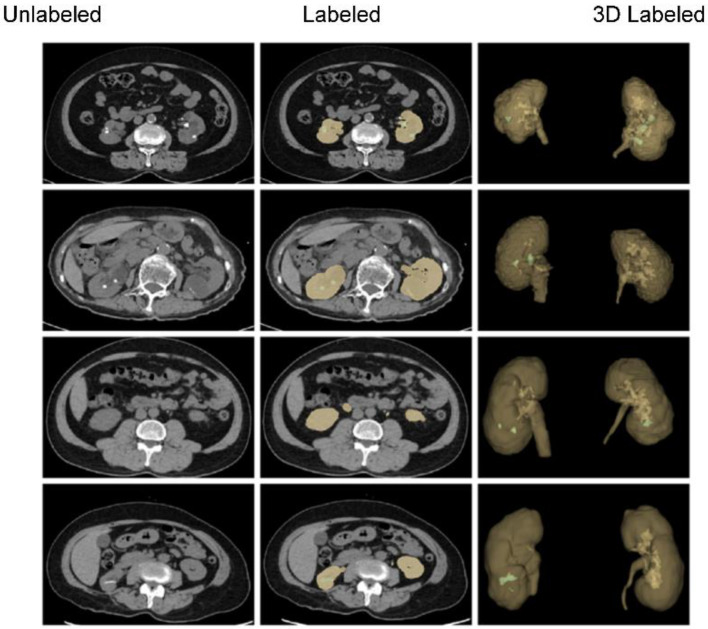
A few of the samples in both 2D and 3D from our annotated dataset of unenhanced abdominal CT images (kidney: yellow; kidney stone: green).
